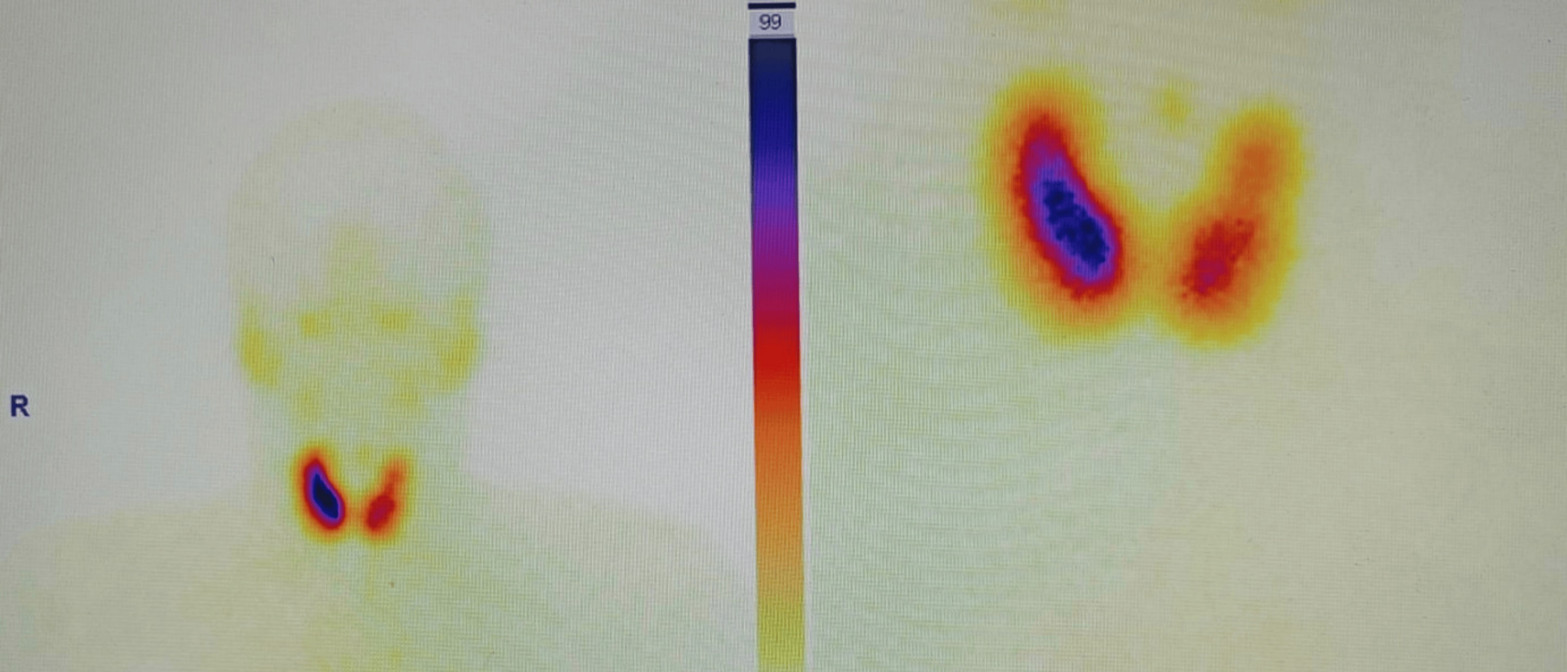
Thyroid scintigraphy report
